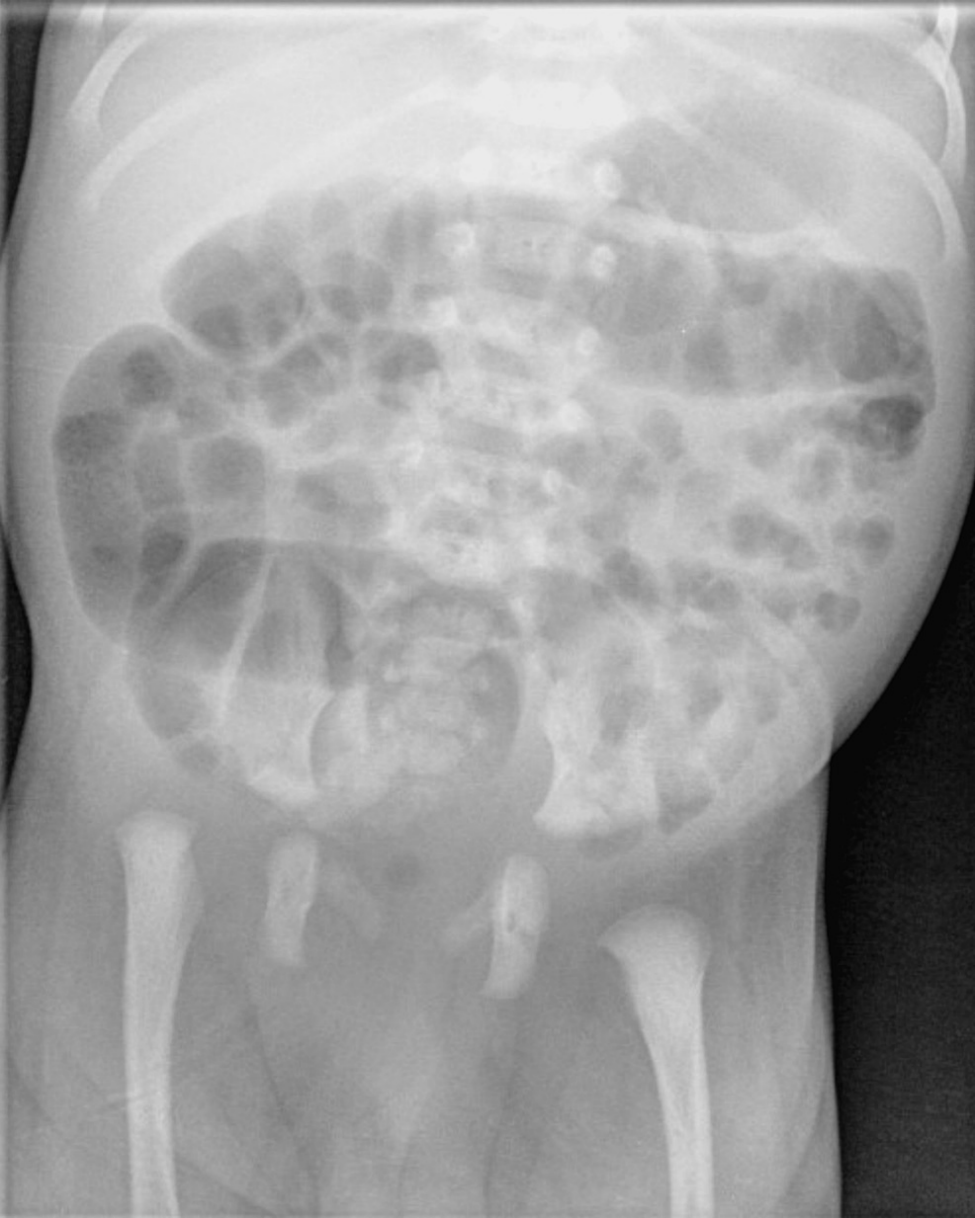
Pelvic radiograph at birth showing right hip joint dislocation
